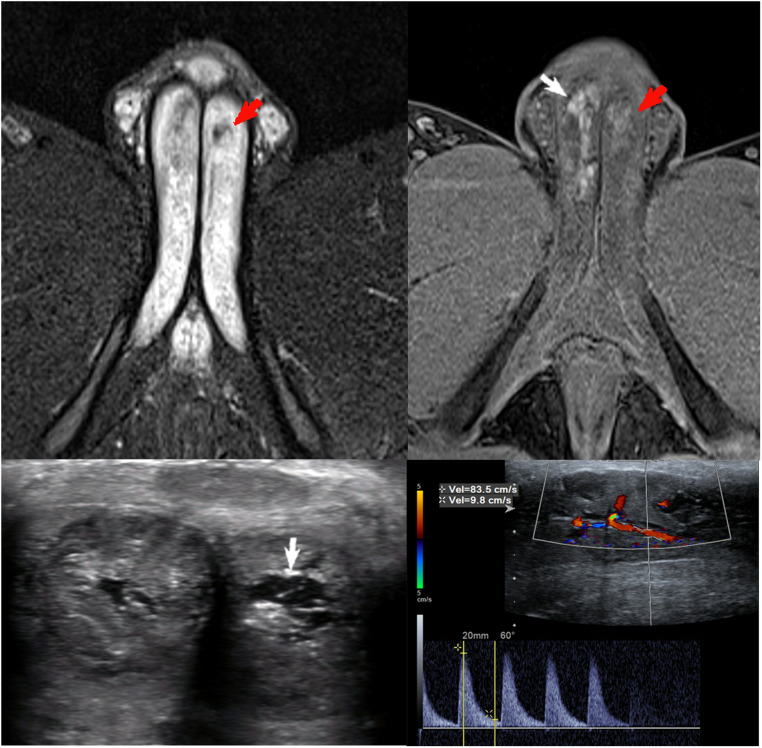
MRI and CDUS images in non-ischemic priapism. Top left – T2w MRI axial section through the crura/proximal corporal cavernosa showing ill defined low T2 signal in the central corpora (red arrow), this likely represents contused tissue with hematoma. Top right – T1w FS post contrast arterial phase axial section at the same position as the adjacent image. Arrow indicating the early enhancement and contrast blush at the site of arterio-venous fistula (red arrow). A similar contralateral blush is also seen indicating bilateral injury (white arrow). Bottom left – axial ultrasound image at the same level showing an ill-defined hypoechoic region in the central corpus cavernosum indicating the sac of the AVF (white arrow). Bottom right – longitudinal image of the left corpus cavernosum showing characteristic low resistance waveforms (PSV 83.5 cm/s, EDV 9.8 cm/s) indicative of an arteriovenous fistula.
